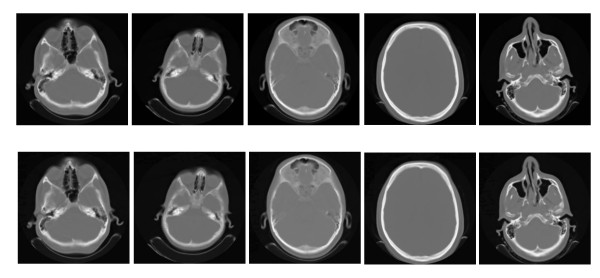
The original (top row) and watermarked image (bottom row) in contourlet domain for several CT scans.
